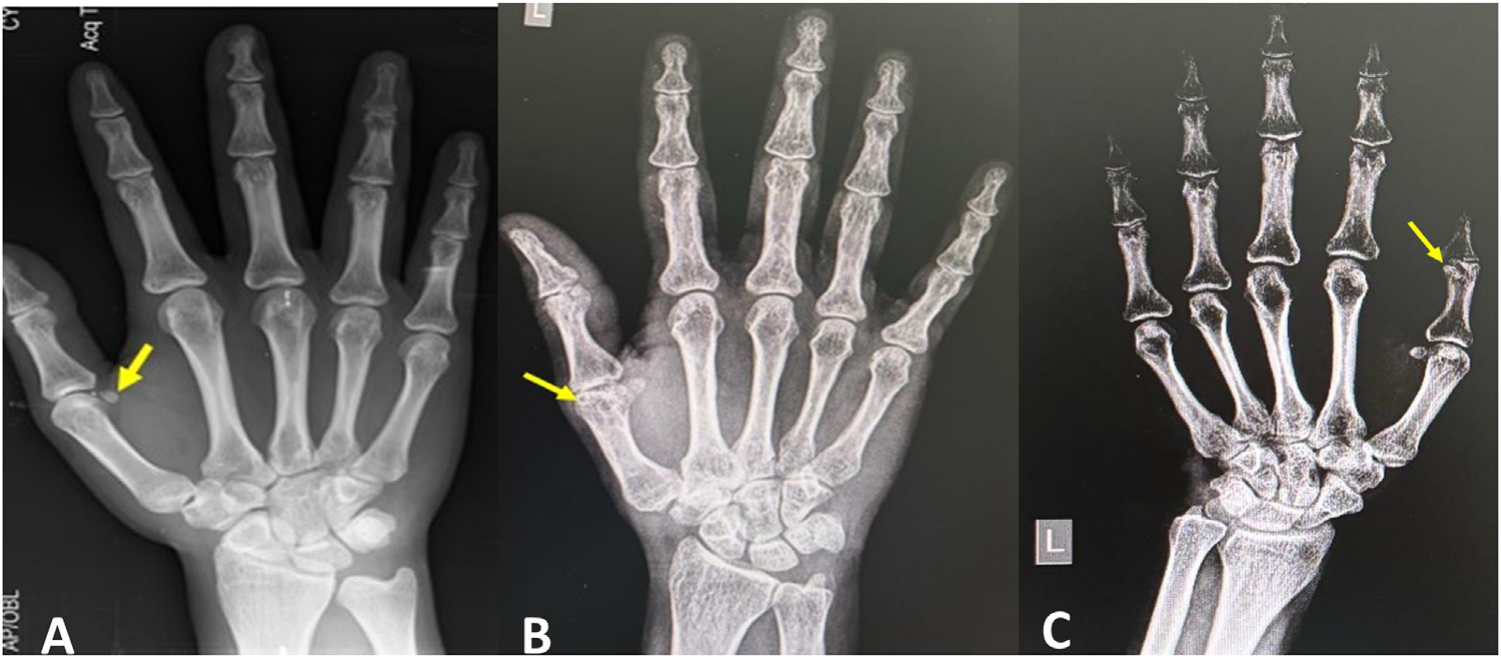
Plain radiographs (anteroposterior views) of the hand showing the sesamoid bones (marked by the arrows), which are observed at the thumb (first digit); (A) sesamoid bone at the medial aspect of the first metacarpophalangeal joint; (B) sesamoid bone at the lateral aspect of the first carpometacarpal joint; (C). sesamoid bone at the interphalangeal joint.
